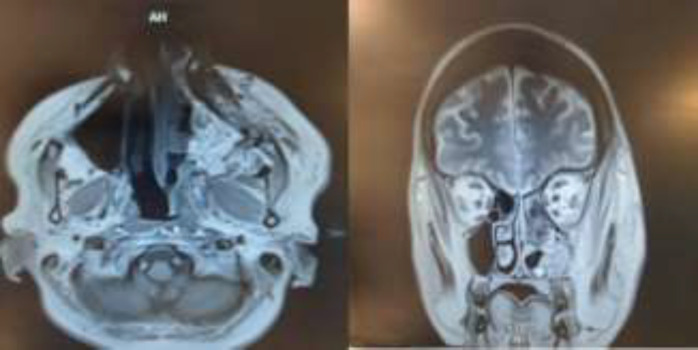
Magnetic resonance imaging findings after the intervention
